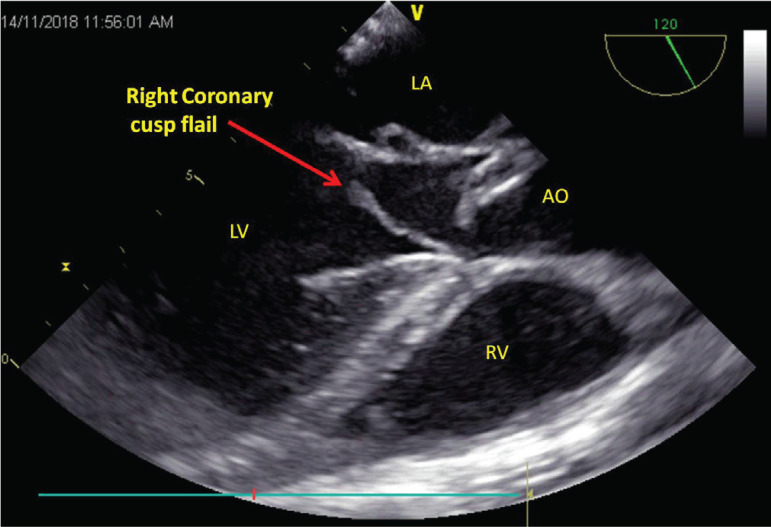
Transesophageal echocardiography showing right coronary cusp flail causing perforation of anterior mitral leaflet. AO=aorta; LA=left atrium; LV=left ventricle; RV=right ventricle
